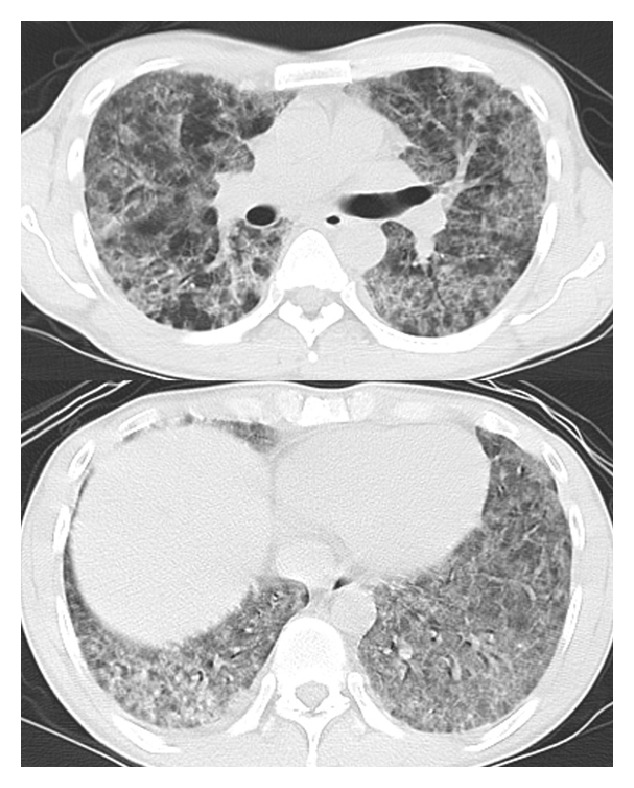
Chest computed tomography revealed bilateral airspace consolidation and ground-glass opacity.
